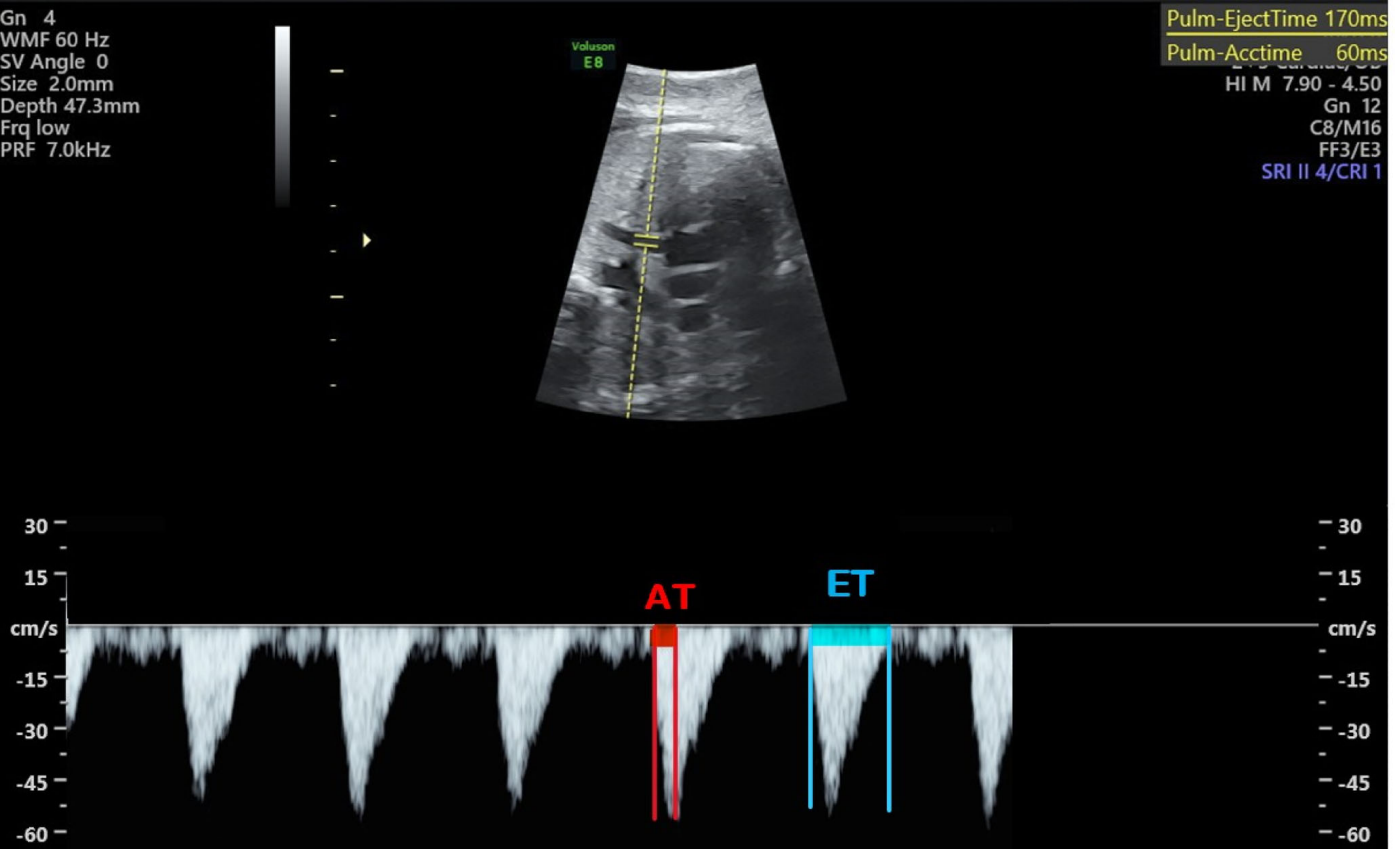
Left pulmonary artery Doppler parameter. AT stands for acceleration time, which is the time between the start of systole and its peak, and ET stands for ejection time, which is the time between the start of systole and its end.
